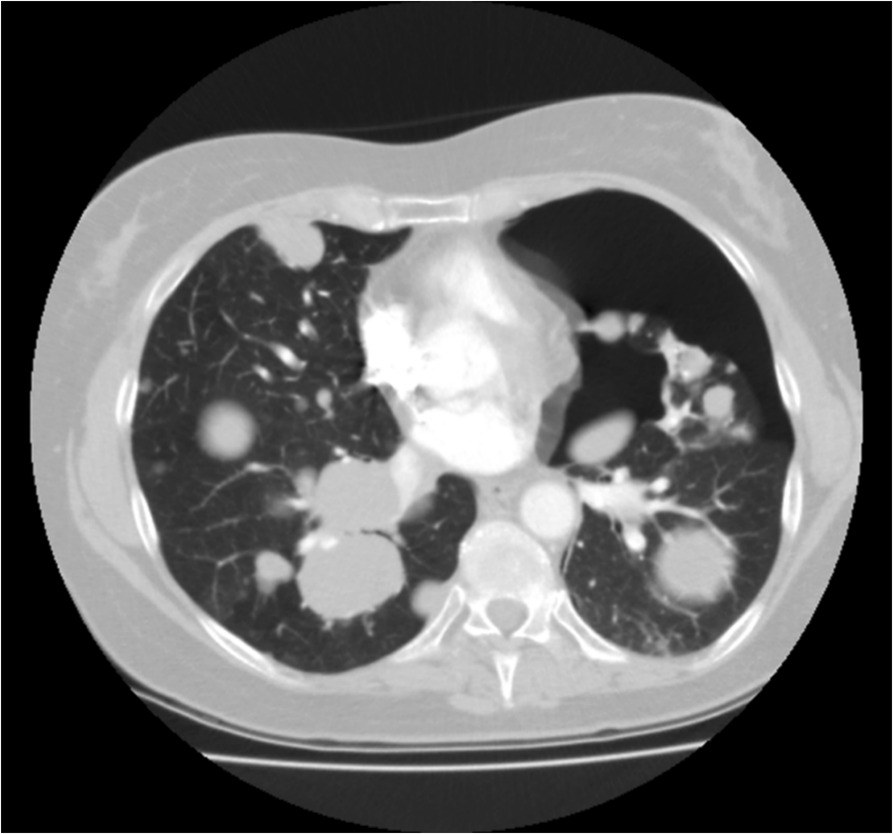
CT scan of patient 4. The CT scan shows the massive pulmonary metastases and a left-sided pneumothorax.
